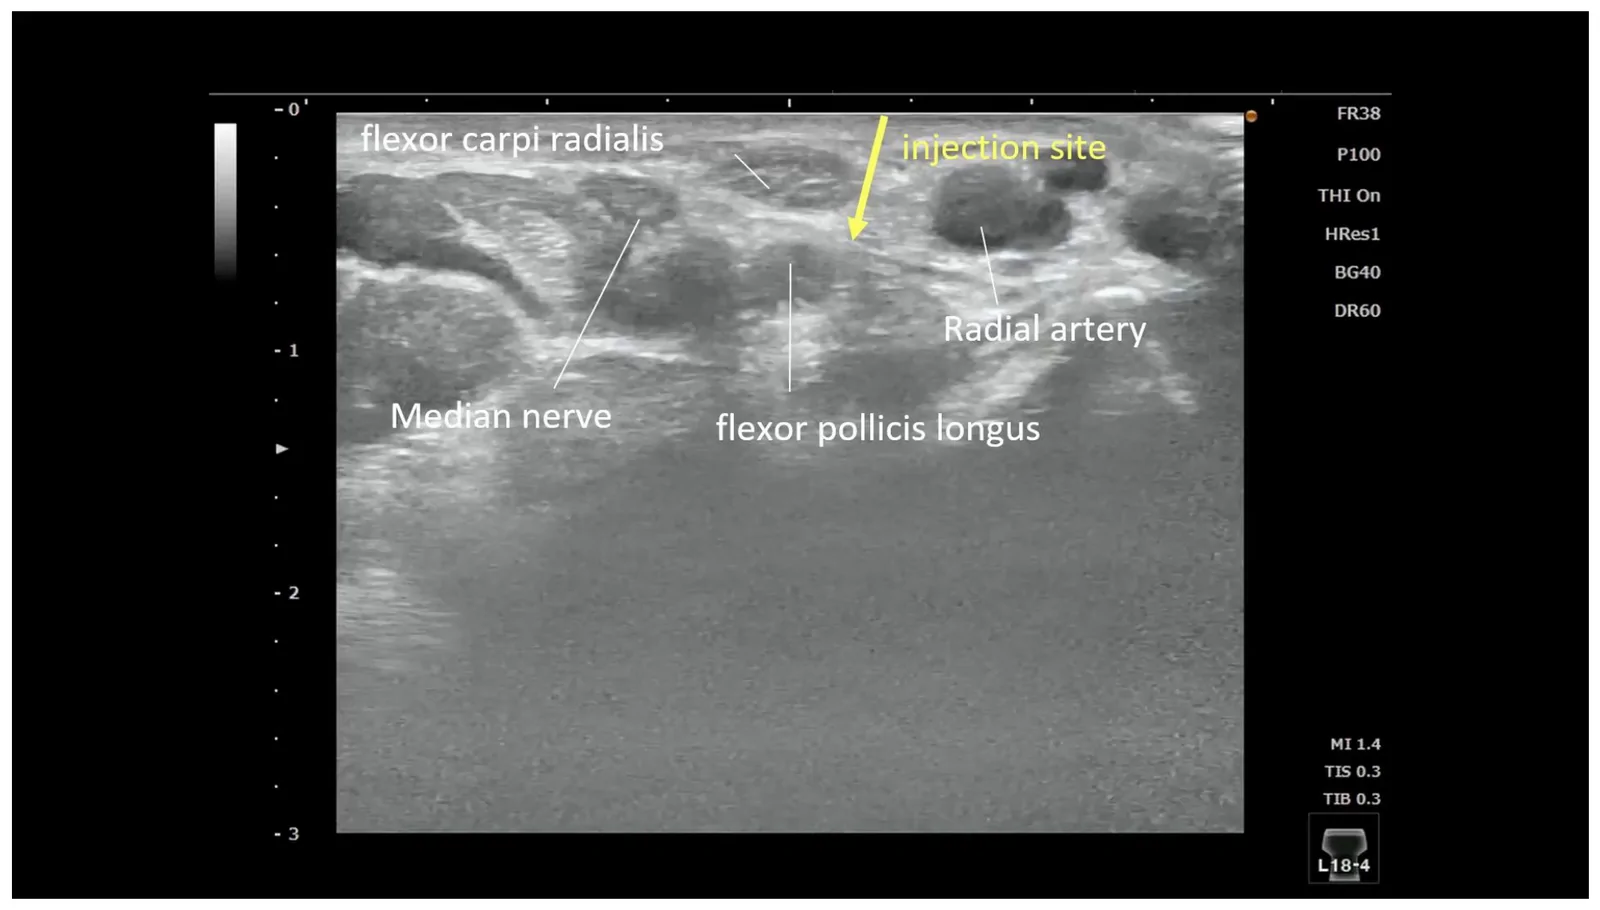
Point 13—median nerve/FPL/FCR interface: ultrasound-guided fascia hydrorelease; yellow arrow indicates the injection site.
